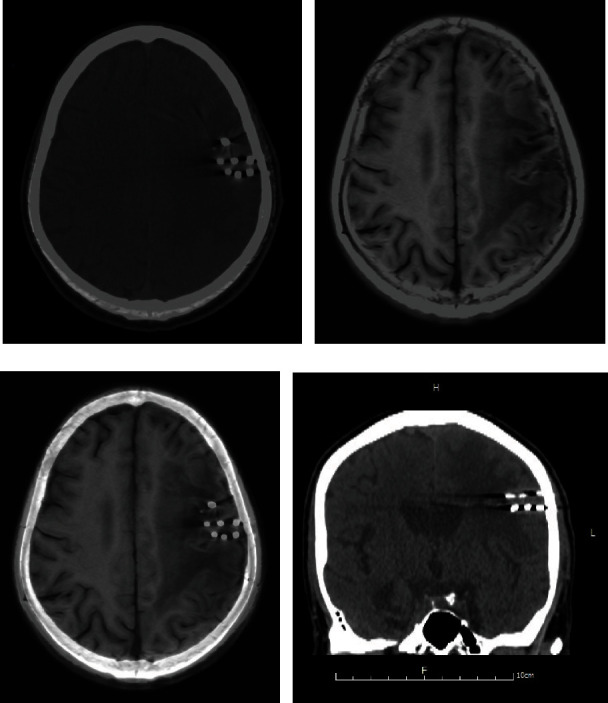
A case to show the same seeds identified on different types of images. (a). CT image; (b). MR T1 FLAIR image; (c). CT-MR fusion image; (d). Reconstructed image along needle path, used to confirm reference seeds.
